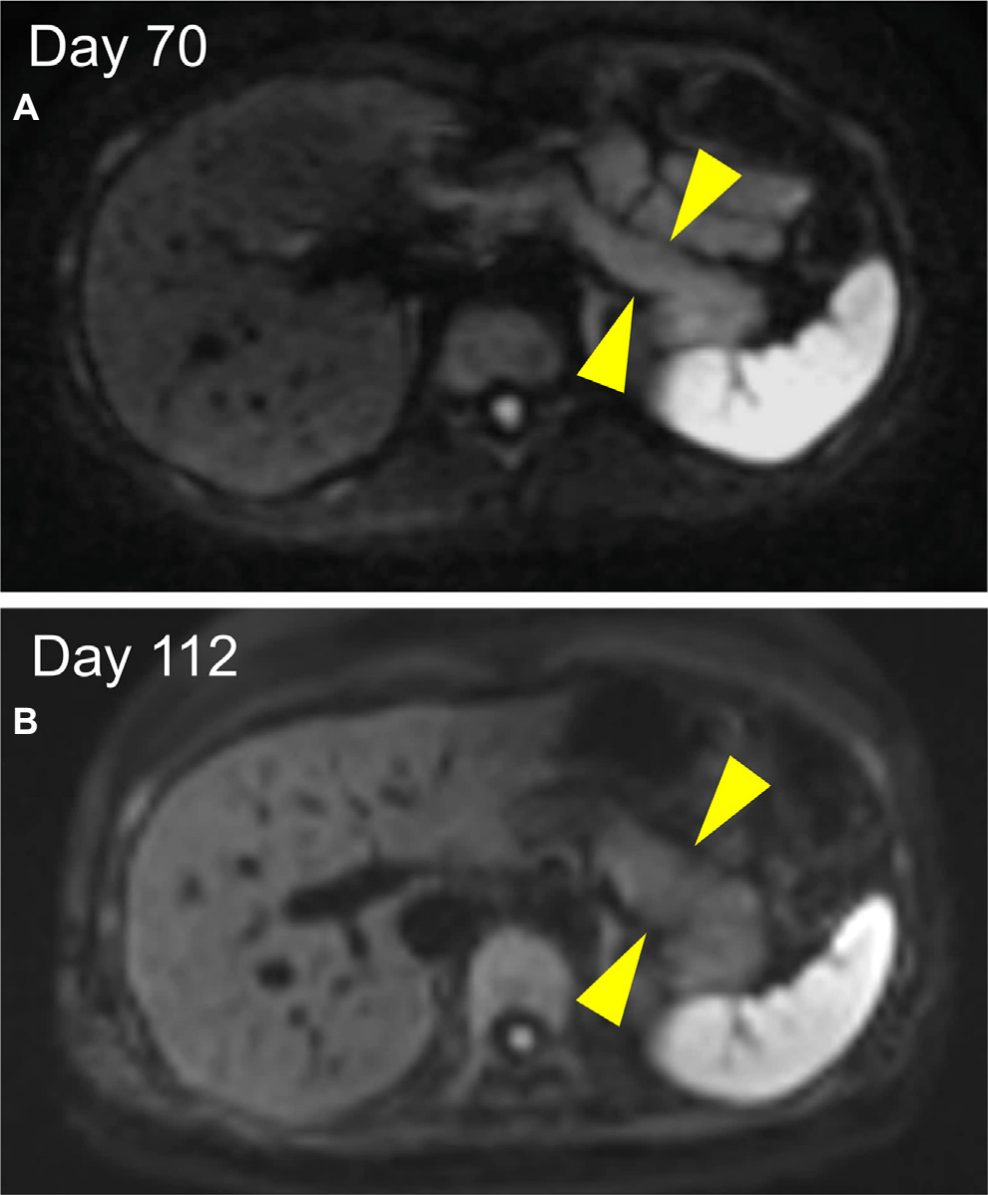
DWI images after treatment introduction. (A and B) DWI images on day 70 and 112. Yellow arrowheads show the pancreatic body and tail with high intensity area.
